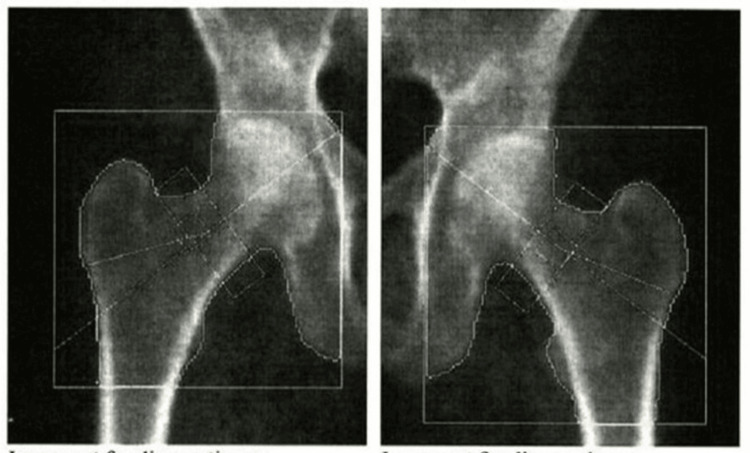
Bilateral hip DXA scans Right and left hip DXA scan images were obtained using a Hologic densitometer (Hologic Inc., Marlborough, MA, USA). The right hip is shown on the left side of the image and the left hip on the right, in standard radiologic convention. Quantitative DXA measurements are presented separately in Table 3. DXA, dual-energy X-ray absorptiometry
